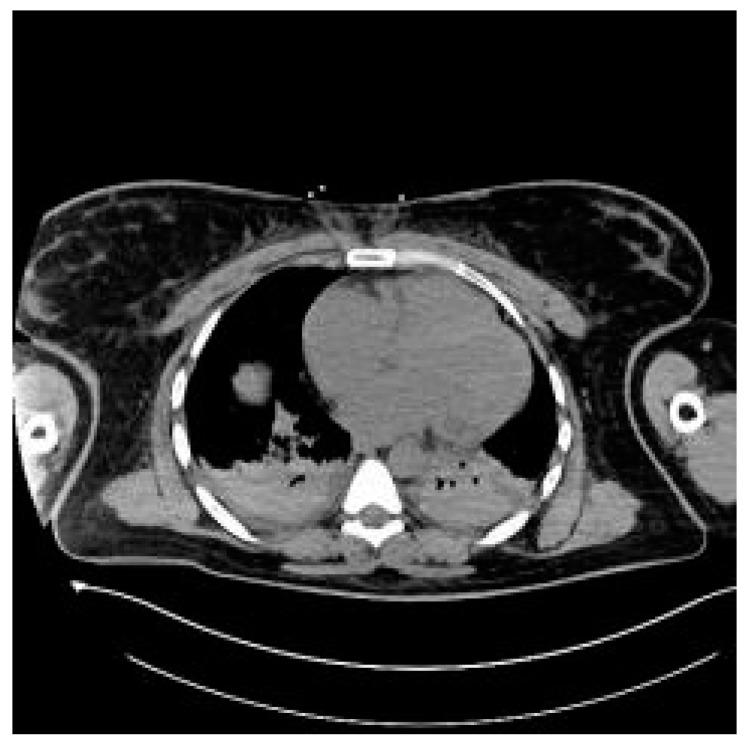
Chest CT with lung consolidations bilaterally.
